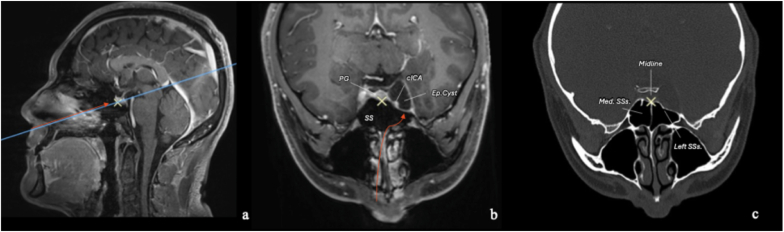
Preoperative radiological planning of the contralateral mononostril endonasal approach. Planning was performed using Carestream Image Suite software, with spatially synchronized images. The blue line in (a) indicates the orientation of images (b) and (c). The yellow cross is co-registered across all three images and identifies the pituitary gland (PG). The orange arrow in (a) and (b) indicates the planned surgical trajectory. The cyst creates a natural surgical corridor within the lateral compartment of the cavernous sinus (CS), between the cavernous internal carotid artery (cICA) and the lateral wall of the CS. (c) A CT scan in the bone window is essential for understanding sphenoid sinus (SS) anatomy. In this case, the median septation identifies the sella and thus the midline, while a smaller left septation (*better visualized in*Fig. 6) inserts onto the left ICA prominence. Based on this anatomy, a contralateral transrostral approach to access the left CS can be performed without the need for a complete sphenoidotomy, preserving the right sphenoid sinus.
